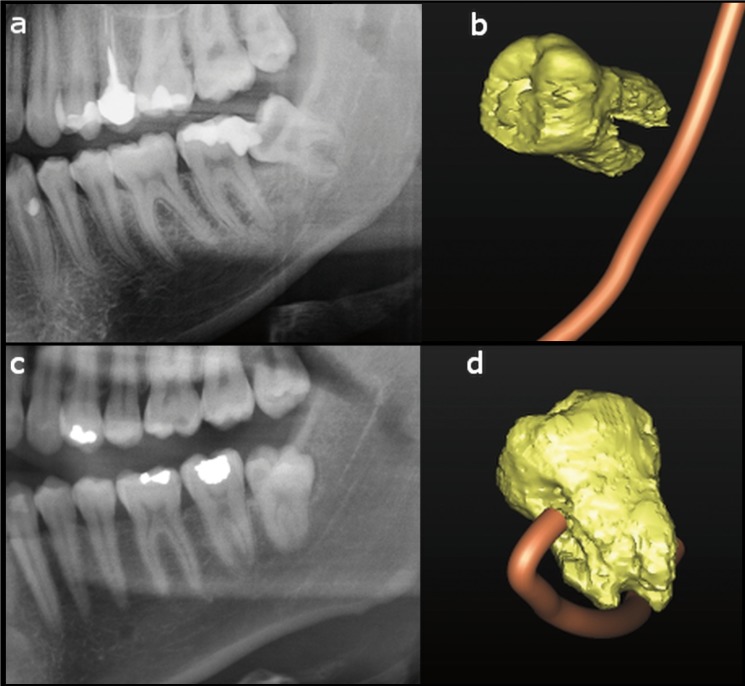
Panoramic radiography magnification of a lower left third molar in a male subject (a). The apex of the root is superimposed on the MC. The CBCT 3D reconstruction of (a) shows no contact between the tooth and the mandibular canal (b). Panoramic radiography magnification of a lower left third molar in a young woman (c). The apex of the root is superimposed on the mandibular canal. In this case the CBCT 3D reconstruction confirm a close contiguity between the tooth and the MC (d).
